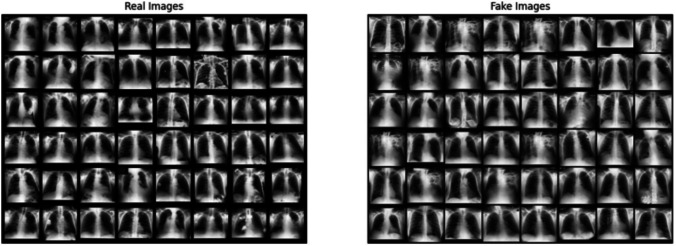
Real and fake frontal chest radiographs. The real frontal radiographs served as the training dataset for the discriminator, while the fake radiographs were generated by the generator. Through competition during training, the generator learned to create images resembling realistic data
